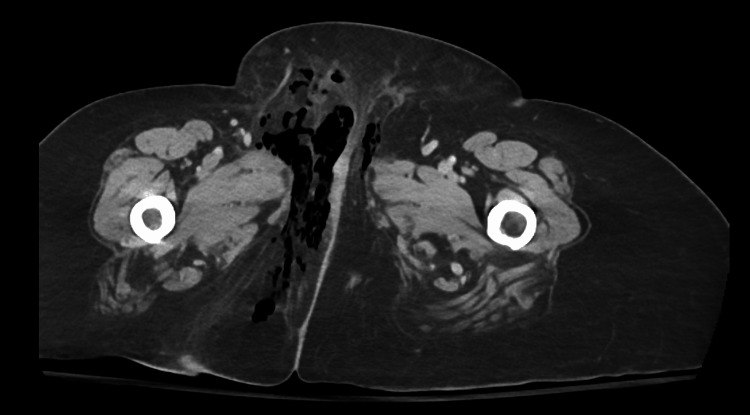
CT (axial view) with contrast of the abdomen and pelvis CT with contrast of the abdomen and pelvis showed extensive subcutaneous gas collections in the right labia, right adductors, and perineum.
